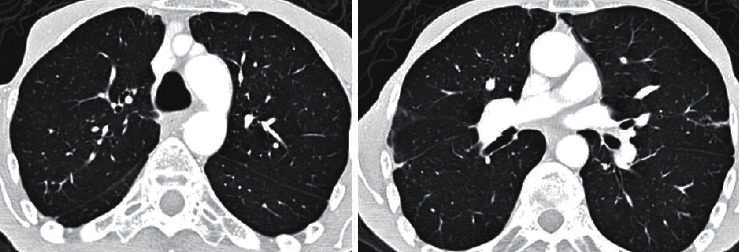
Thorax CT performed in 2016. There is no apical fibrotic changes and traction bronchiectasis in the upper zone of the right lung.
